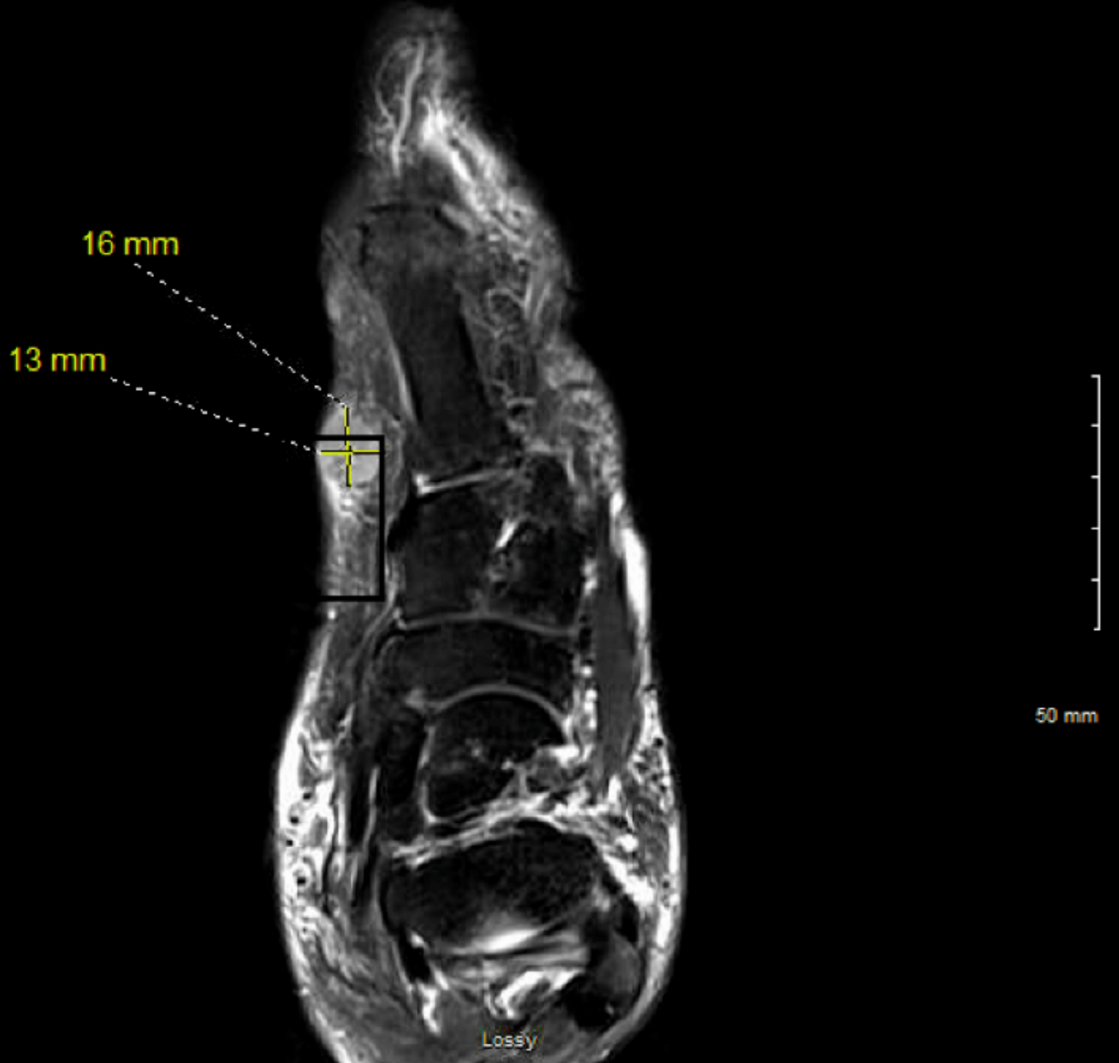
Left foot MRI (February 7, 2023) showing a 13 x 16 mm lesion on the plantar surface
